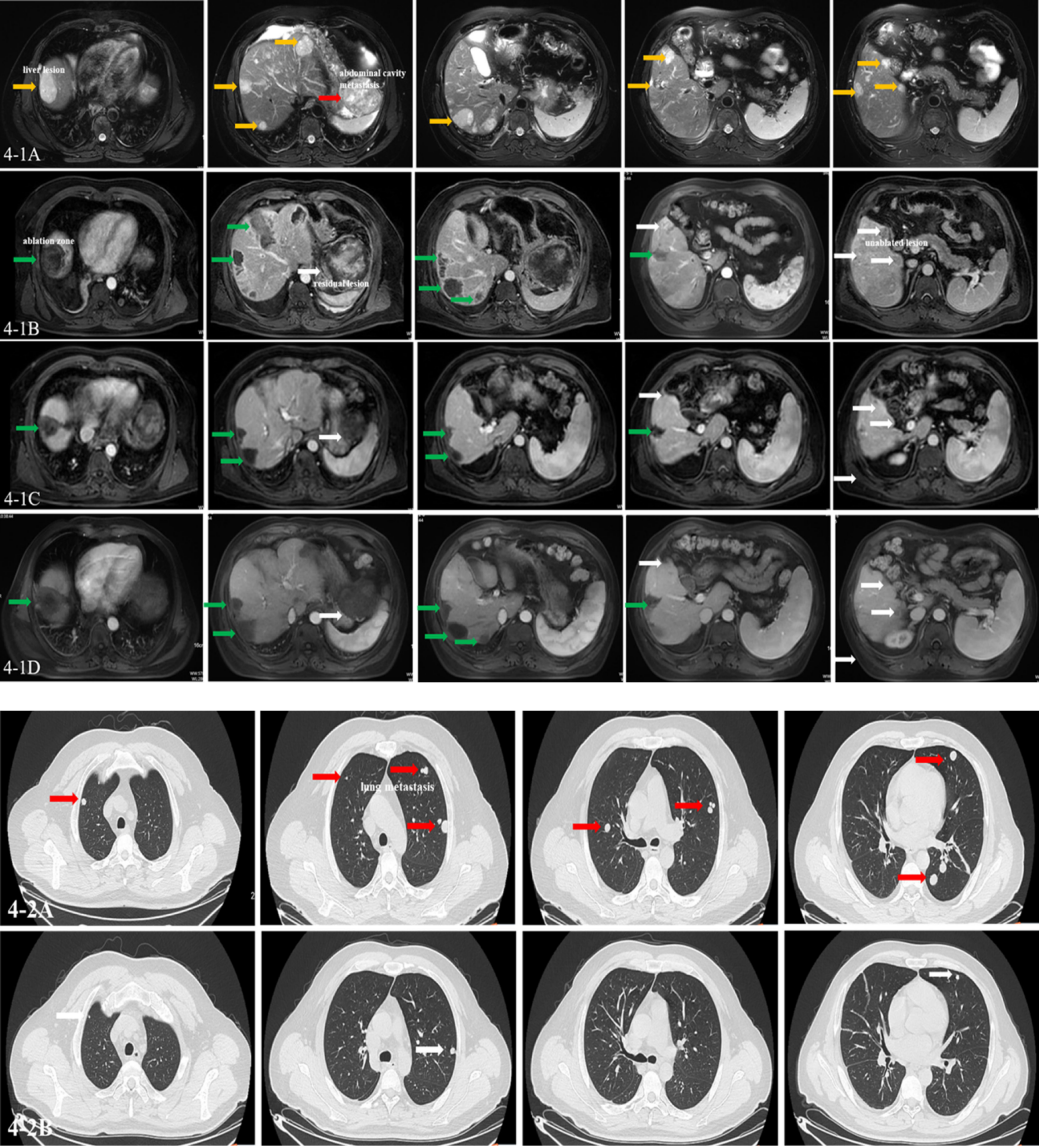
Imaging of clinical events. A man aged 45 years was diagnosed as having moderately differentiated hepatocellular carcinoma at Barcelona Clinic of Liver Cancer (BCLC) C stage, who received the combination therapy. (4-1A) The T2 phase of MRI images showing multiple lesions in the liver and abdominal cavity (yellow arrow: liver lesions; red arrow: abdominal cavity metastasis). (4-1B) The arterial phase of MRI images showing multiple ablated zone (green arrow), subtotal ablation of the lesions, and unablated lesion in the liver (white arrow) at baseline. (4-1C, D) The arterial phase of MRI images showing multiple ablated zone (green arrow) with no enhancement and the evaluated lesion shrunken with blood supply reduced (white arrow) after 6 and 12 cycles of combination therapy. The effect was evaluated as partial response (PR) by modified Response Evaluation Criteria in Solid Tumors (mRECIST). (4-2A) CT images showing multiple metastasis in the lung at baseline (red arrows). (4-2B) CT images showing lung metastasis shrunken obviously and some disappeared after six cycles of combination therapy (white arrow). The effect was evaluated as PR by mRECIST. Until now, the patient is alive with the imaging evaluated as stable disease (SD) lasting for 18 months.
